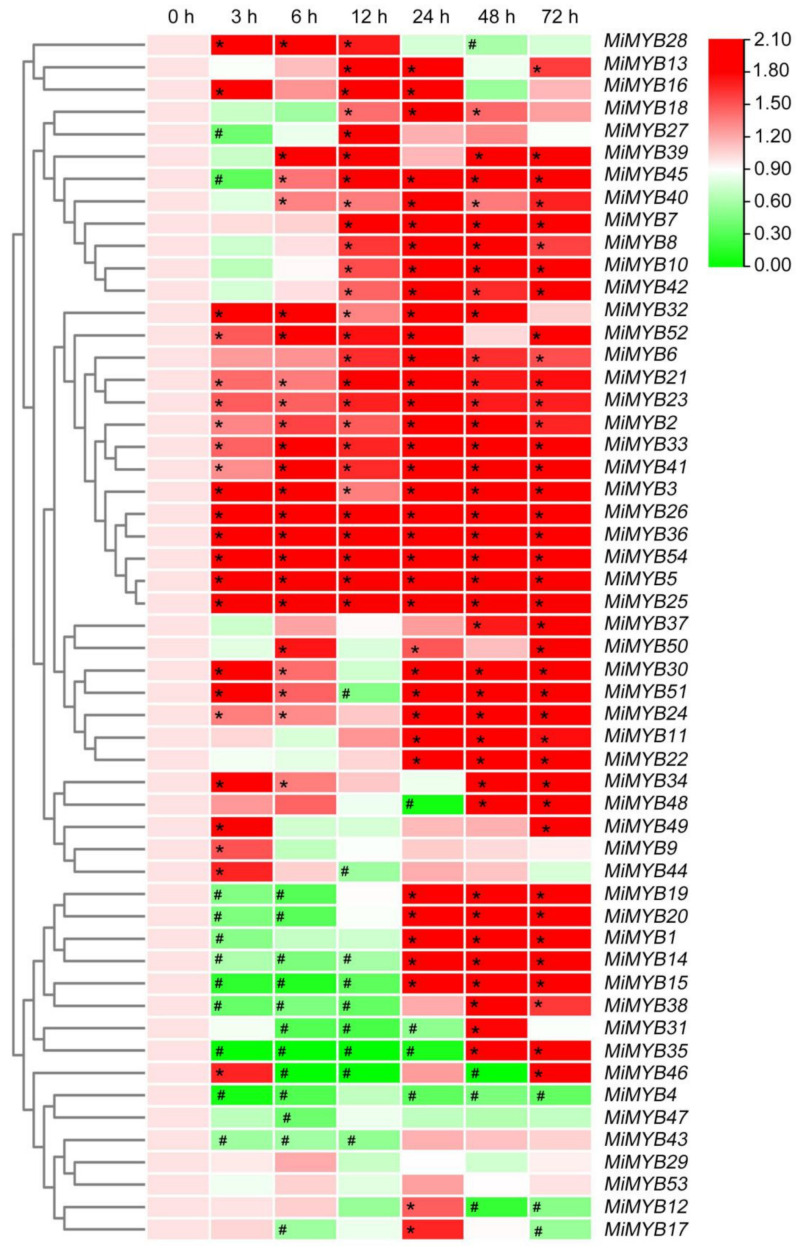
Expression patterns of *MiMYBs* in response to *X*. *campestris* pv. *mangiferaeindicae* infection. Relative transcript levels of *MiMYBs* with 2 × 10^7^ CFU/mL *X*. *campestris* pv. *mangiferaeindicae* infected mango leaves by spray at 0, 3, 6, 12, 24, 48, and 72 h. The relative transcript levels of *MiMYBs* in the non-sprayed mango leaves (0 h) were normalized to 1.0. The color scale represents gene expression levels with high transcript levels (red) or low transcript levels (green). The calculation method was 2^−∆∆CT^, and ‘*’ means the value exceeds 1.5, while ‘#’ means the value is below 0.5.
